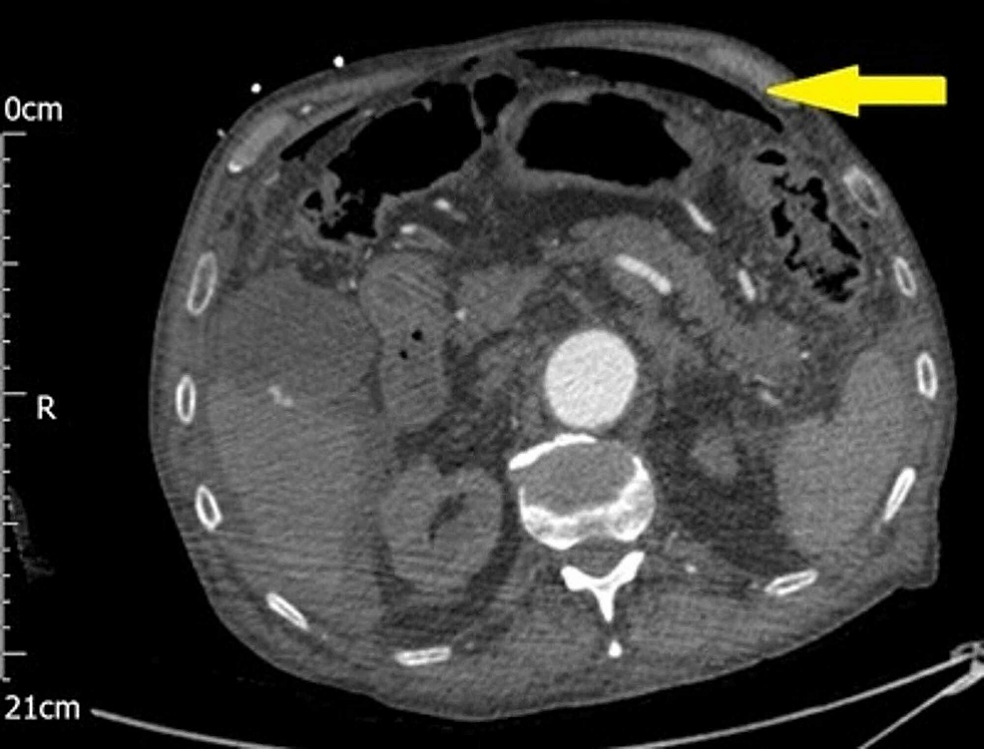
CT abdomen axial view shows free intra-peritoneal air (yellow arrow)
